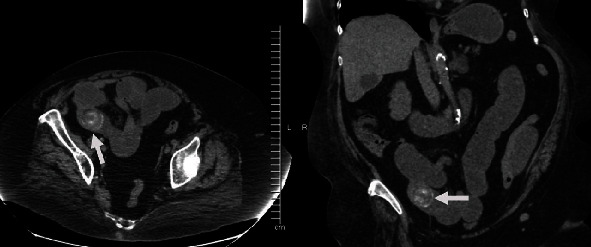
CT scan demonstrating large gallstone (white arrow) causing a small bowel obstruction.
